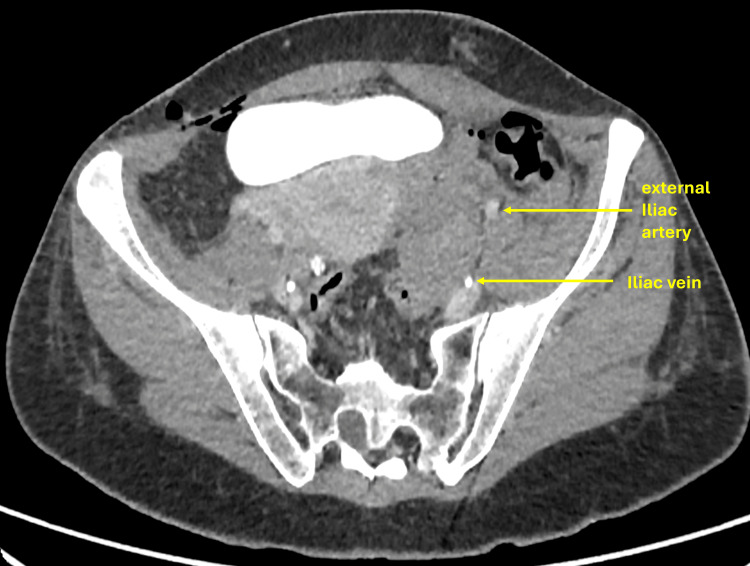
CT of the abdomen showing complete resolution of the hematoma and patent iliac arteries and veins.
